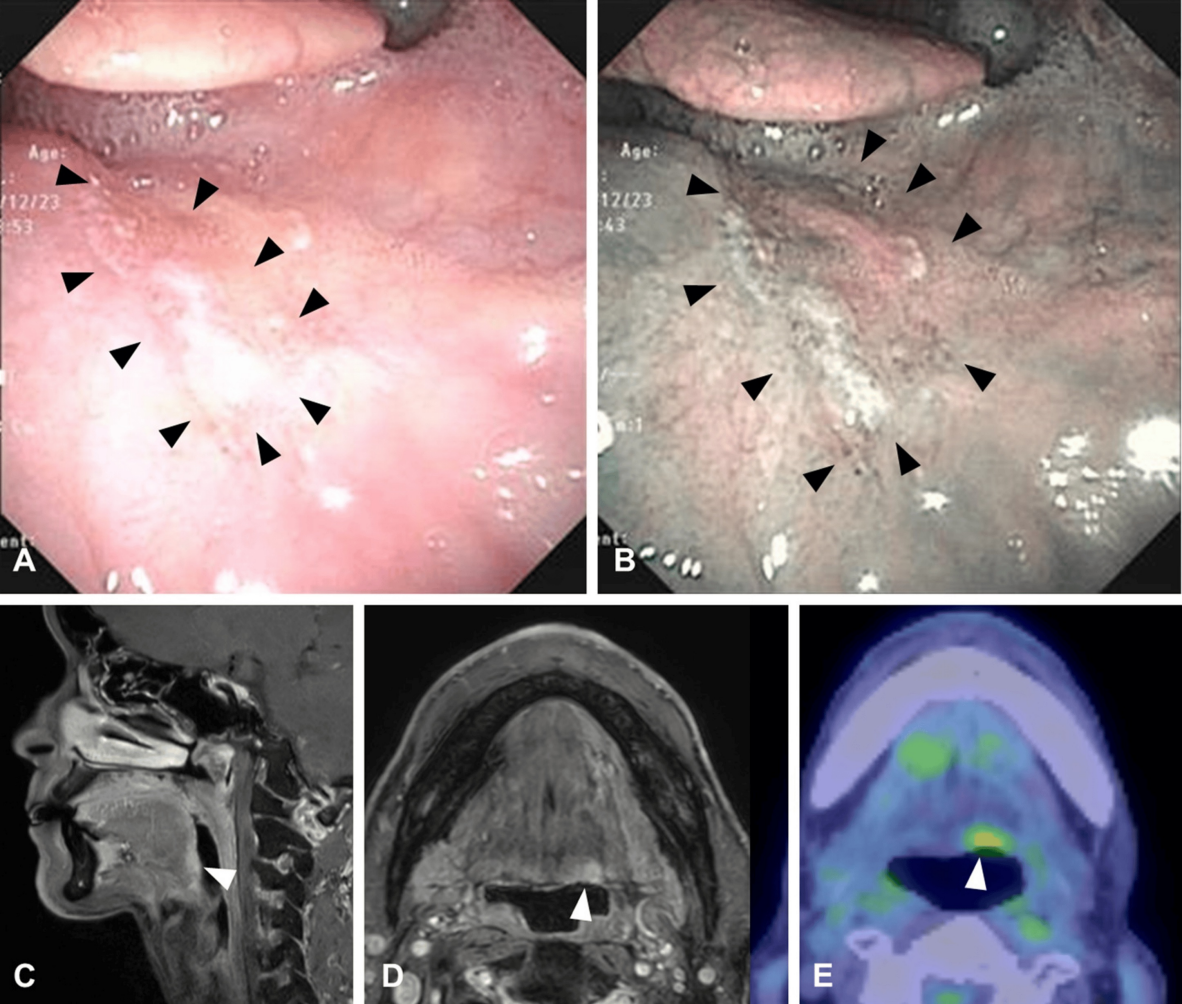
Locoregional findings. A. laryngeal endoscope under normal light. A tumor with an irregular surface approximately 15 mm in size was observed on the left side of the base of the tongue. B. laryngeal endoscope under narrowband optical endoscopy. The surface of the tumor is keratinized, and irregular blood vessels are observed in the surrounding area. C. and D. Gd-enhanced MRI. A contrast-enhanced tumor approximately 1 cm in size was observed at the base of the tongue, and invasion approximately 1 cm deep was suspected. E. PET-CT. FDP accumulation at SUV=3.98 was observed in the same area as contrasted by MRI.
